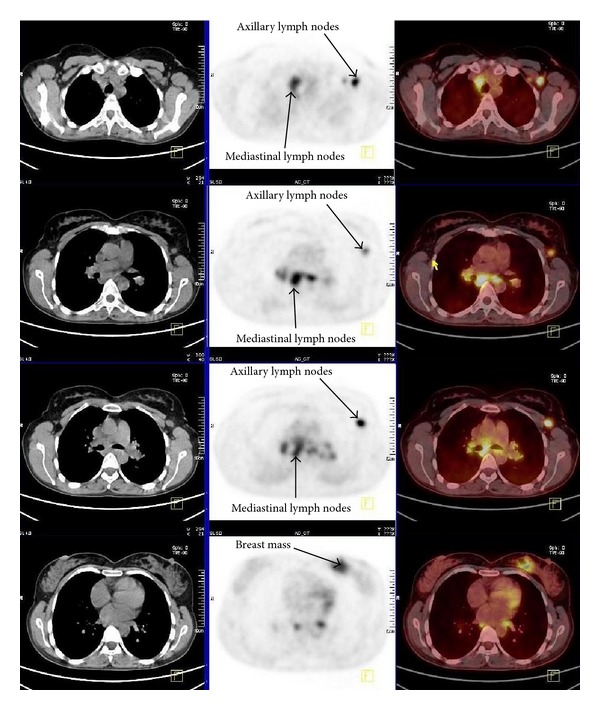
CT (right), PET (middle left), and PET CT (left) transaxial images show intense FDG uptake of mediastinal and axillary lymph nodes and left breast mass.
